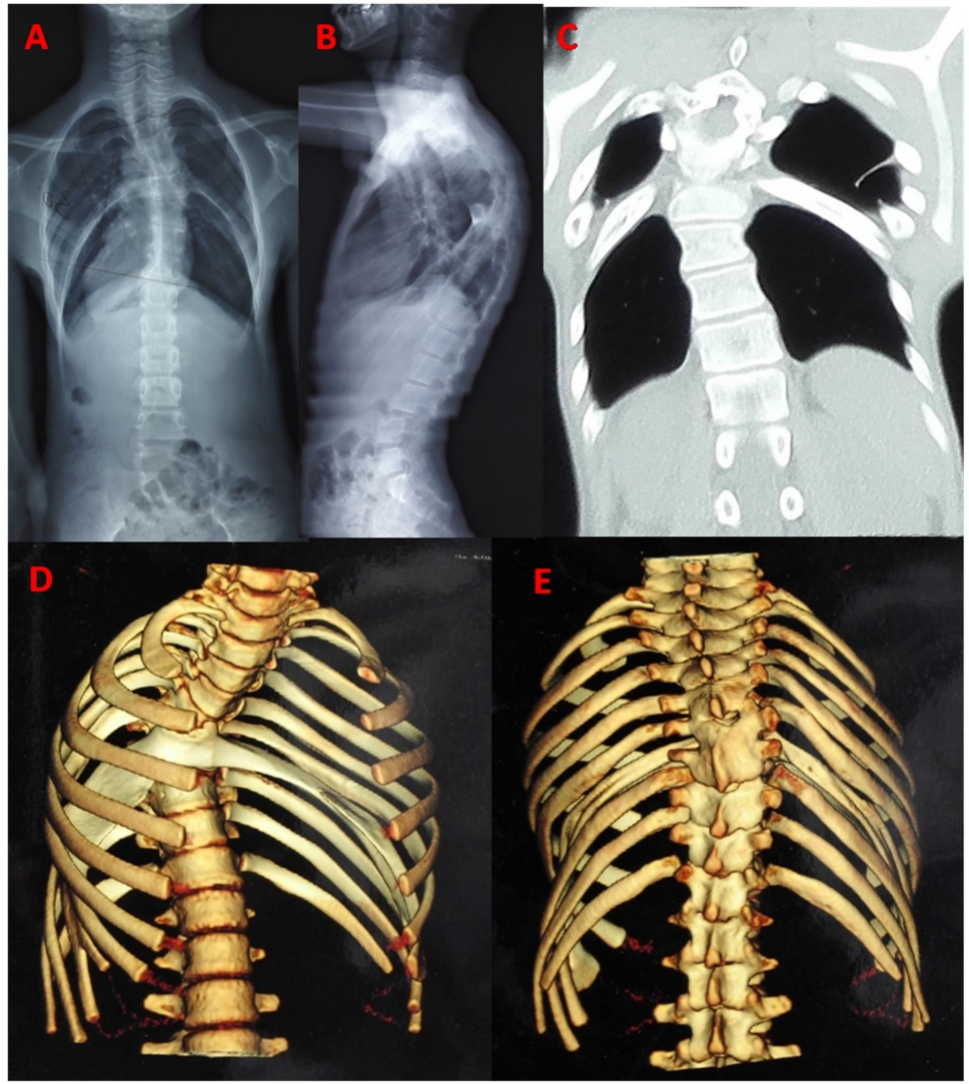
Typical image: a 13-year-old man with bilateral bifid intrathoracic rib. postero-anterior and lateral radiograph showed kyphoscoliosis in thoracic spine, and four thoracic cavity compartments were separated by bilateral intrathoracic rib (A, B). The coronal CT showed two deformed rib extending inferolaterally in bilateral hemithorax, forming focal additional compartment with fiber band-like attachment to pleura (C). CT scan with 3D reconstruction showed bifurcated rib articulated with anterior aspect of the T5 vertebra. Distal anomalous rib had osseous connection with adjacent fused rib, extending bifid rib locally depressed into the thoracic cavity (D, E).
